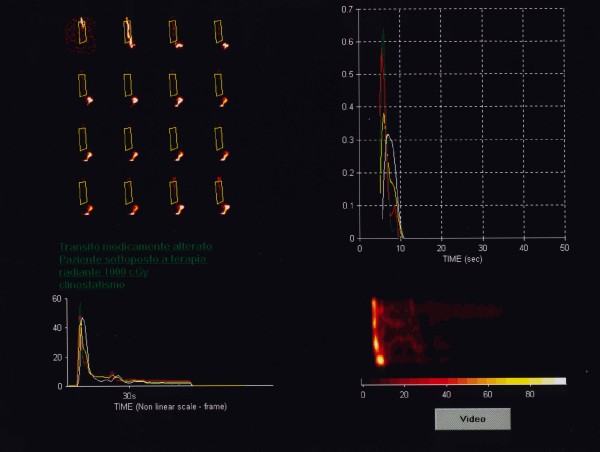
Oesophageal scintigraphy: Dynamic condensed oesophageal images during radiation therapy (slightly altered transit at 10 Gy).
